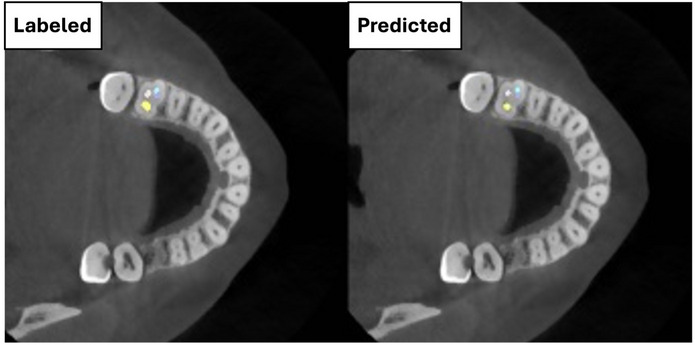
The automatic segmentation of MB2 and main root canals on axial CBCT slices
